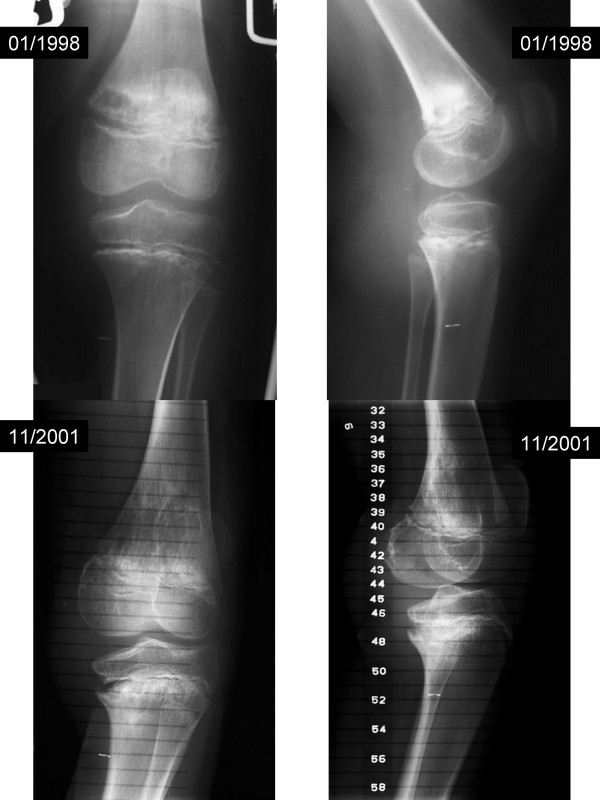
Serial plain films (case one).
